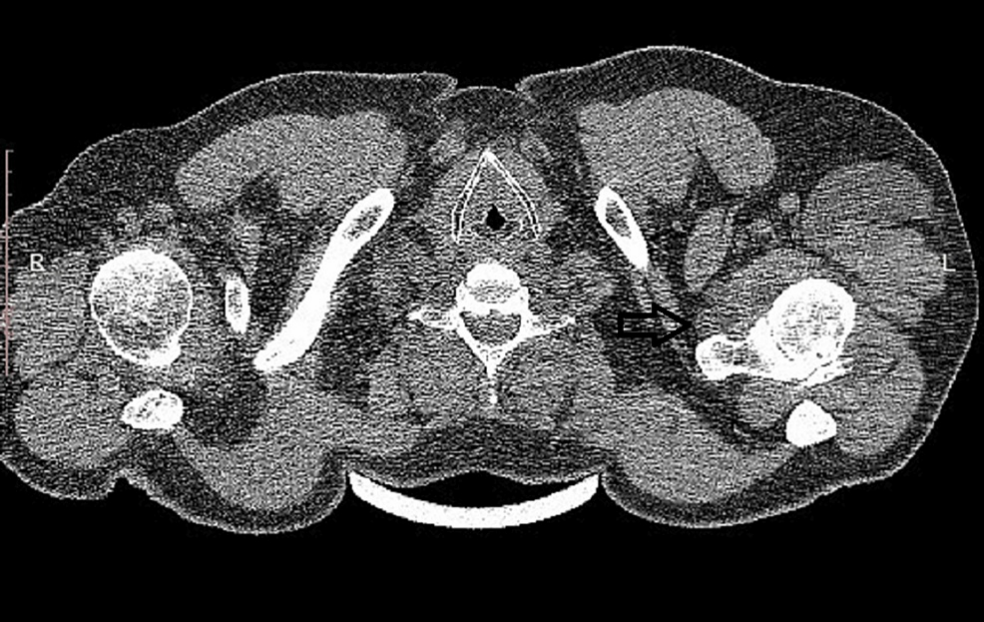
CT image showing the glenohumeral joint, giving rise to the cyst
